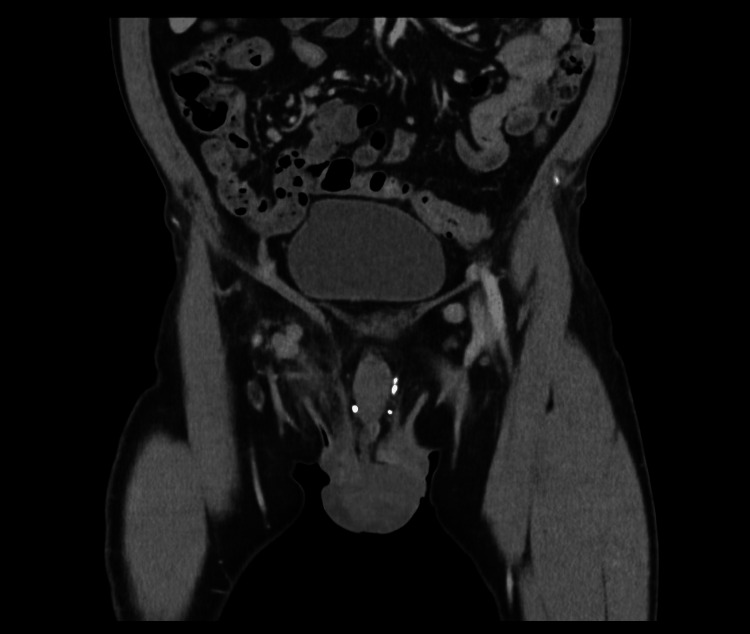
Computed tomography demonstrating soft tissue inflammation in the right groin with prominent inguinal lymph nodes.
